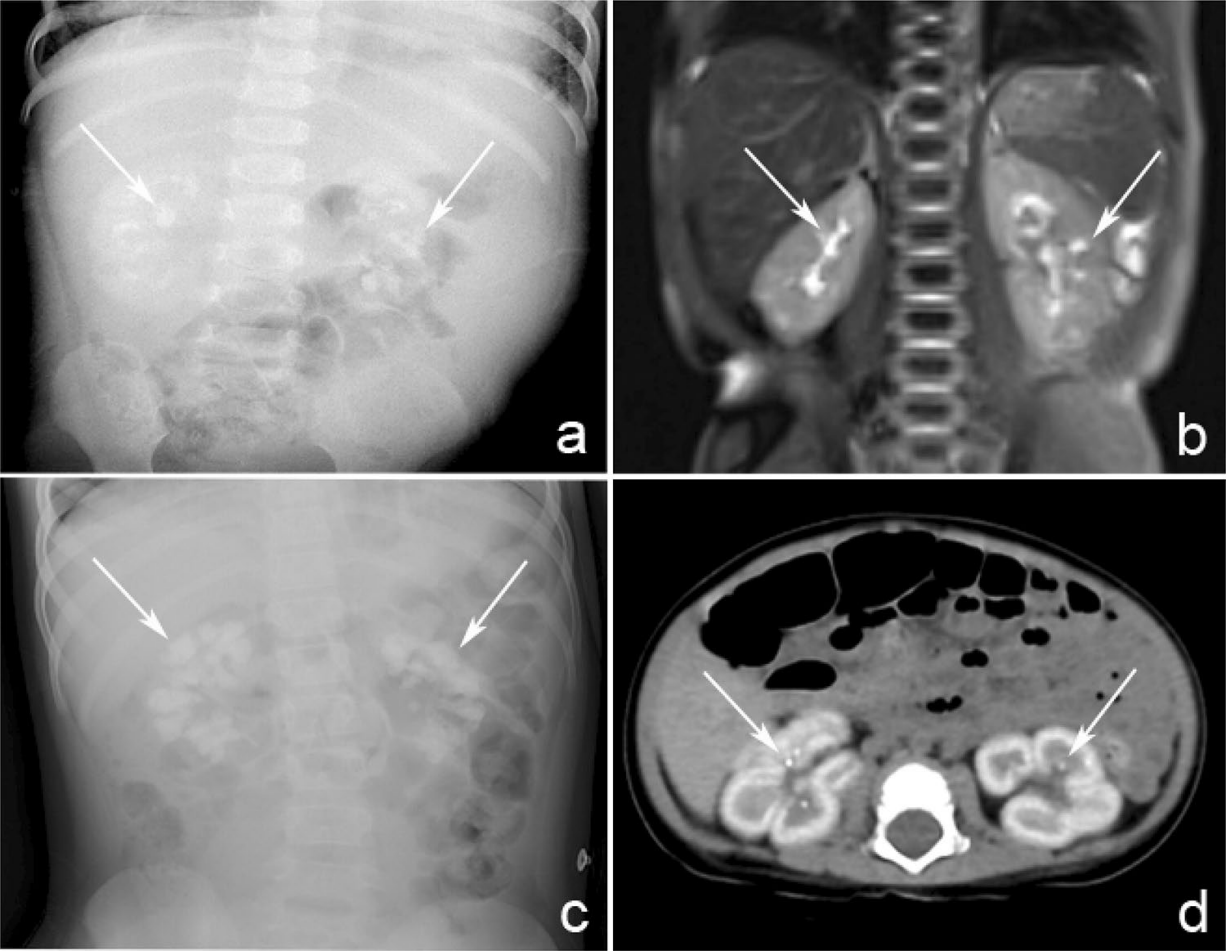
Radiological imaging examinations performed in patients with PH1. a Spot-like high-density imaging was found in bilateral kidneys on X-ray in patient II. b Signal of bilateral renal parenchyma increased diffusely on MRI T2WI in patient III. c The density of bilateral kidneys significantly increased on X-ray in patient IV. d CT scan in patient V showed under illumination of the contour bilateral renal contour, density of bilateral renal cortex significantly increased and multiple spotted high-density imaging in bilateral renal medulla (arrows)
